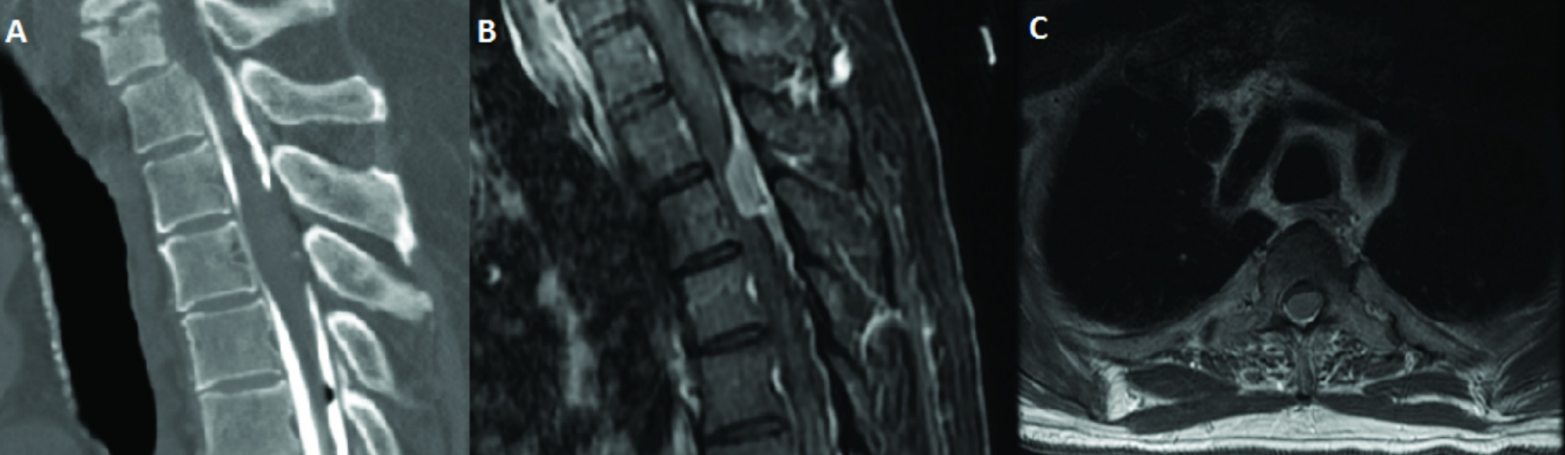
Preoperative imaging. A) Sagittal view of a CT myelogram demonstrating what appears to be an intramedullary lesion at the T3 level; B) Sagittal; C) Axial T1 MRI with contrast of the thoracic spine demonstrating a dorsal, extramedullary dural-based lesion at T3 causing significant canal stenosis and cord compression CT: computed tomography; MRI: magnetic resonance imaging
